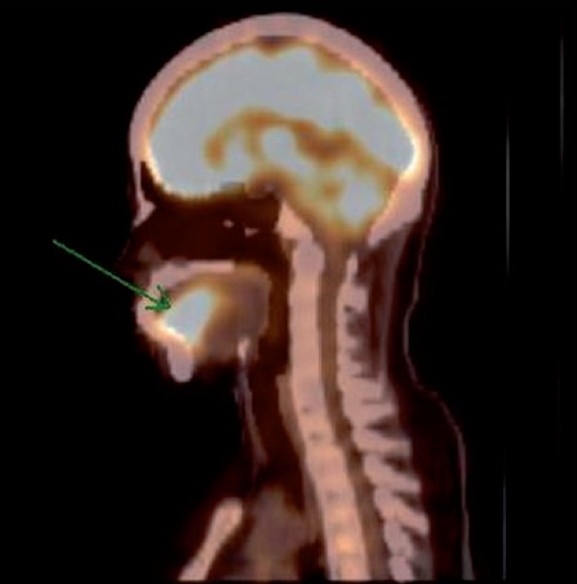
Saggital section of the positron emission tomography-computed tomography fusion image showing intense fluorodeoxyglucose uptake in the tongue
